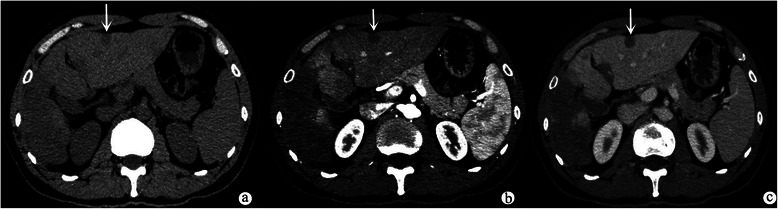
Axial unenhanced CT scan (a) showed lesion (indicated by the white arrow) was homogeneous, hypodense and subcapsular. Axial arterial phase (b) showed the lesion (indicated by the white arrow) was mild homogeneous enhancement. Axial portal vein phase (c) showed the lesion (indicated by the white arrow) was not progressive enhancement
